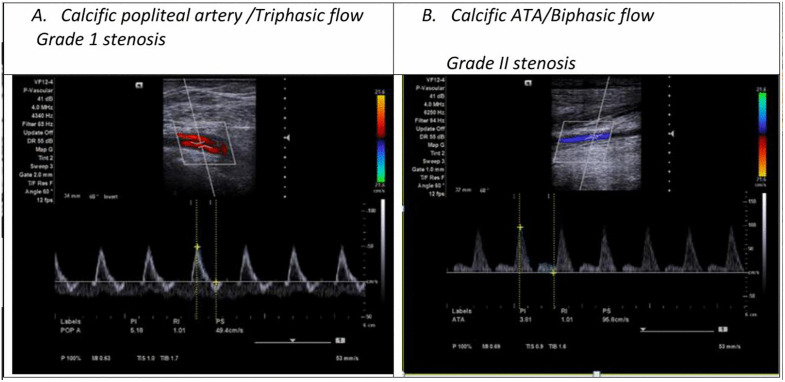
Duplex sonographic selected images extract from the two study participants.
